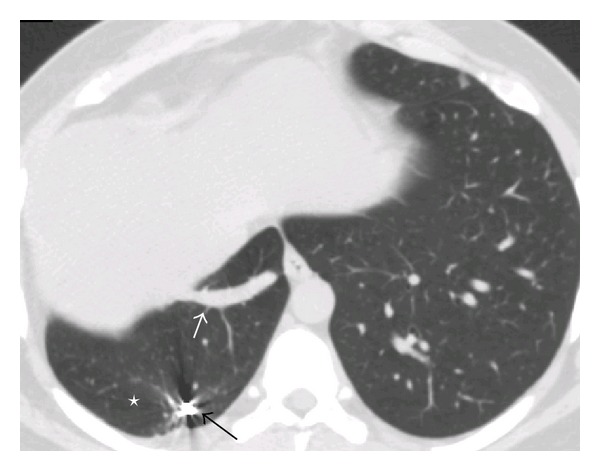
CT axial image depicts complete regression of the sequestration at right posterobasal region (asterix), meandering right inferior pulmonary vein (white arrow). Artefact from the coil within one of the anomalous systemic artery (black arrow).
